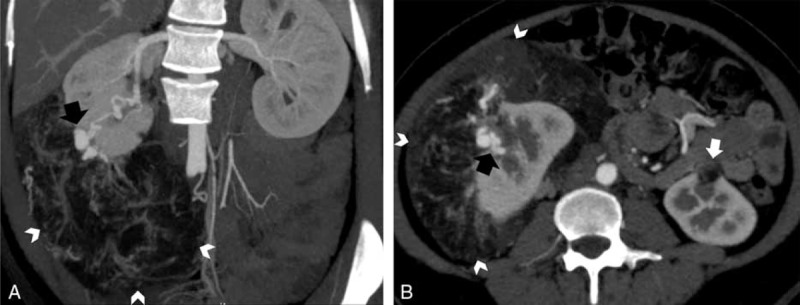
A 28-year-old female with bilateral angiomyolipoma. A, Coronal maximum-intensity projection reformation image shows a large fat-attenuation mass (white arrowheads) arising from the right kidney and a dilated tumoral vessel (black arrow) extending from the mass through the renal parenchymal defect into the renal sinus. B, Axial contrast-enhanced computed tomography also reveals a small fat-containing mass similar to the large one arising from left kidney (white arrow).
